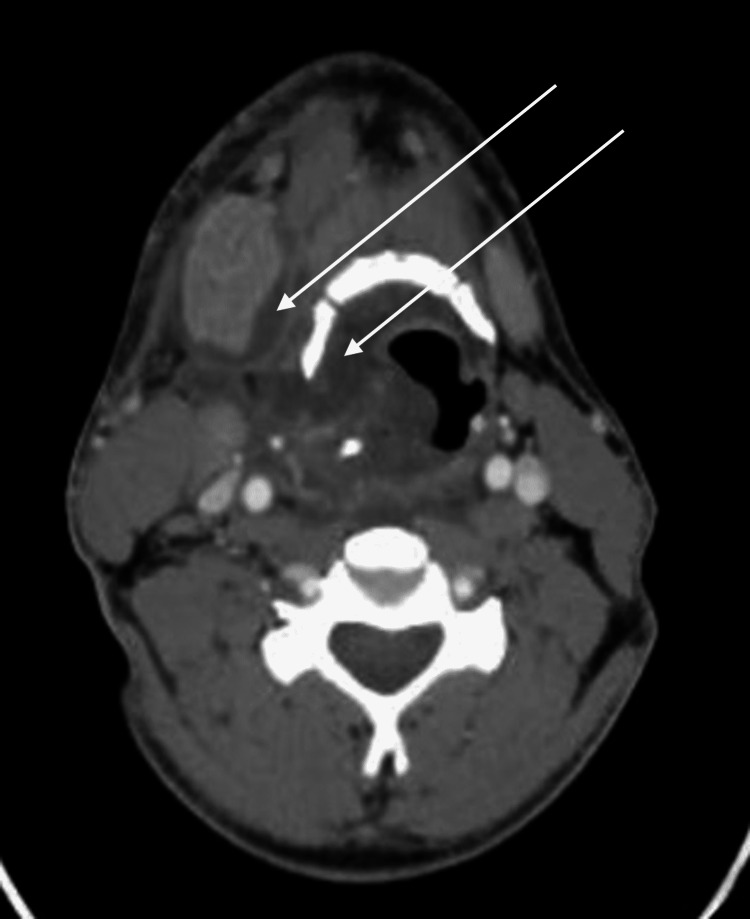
Axial view of CT scan at initial presentation There was significant enlargement and uniform enhancement of the right submandibular gland representing sialadenitis without sialolithiasis with severe surrounding inflammatory changes and mass effect upon the upper aerodigestive tract with severe deviation to the left. Multiloculated fluid (white arrows) was noted within the right aspect of the neck extending into the upper aerodigestive tract in several spaces, including the submandibular, submental, sublingual, paratracheal, and retropharyngeal spaces.
